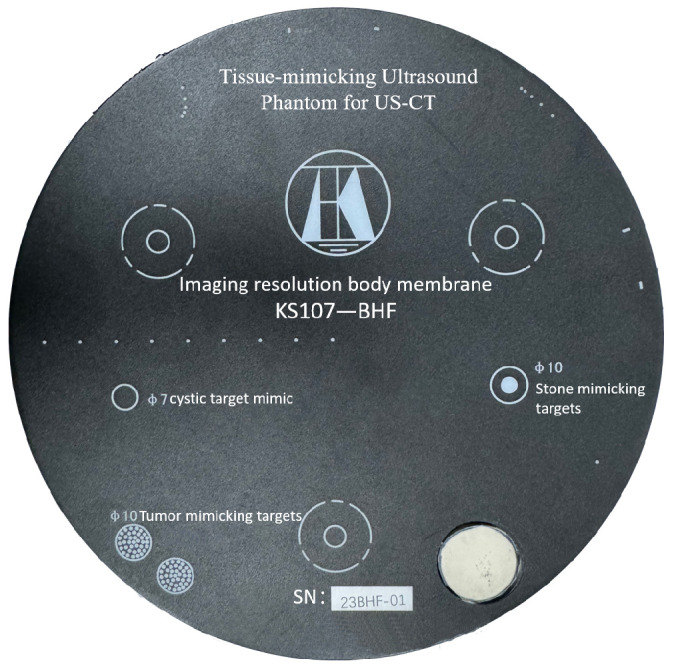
Model KS107BHF resolution ultrasound die body.
